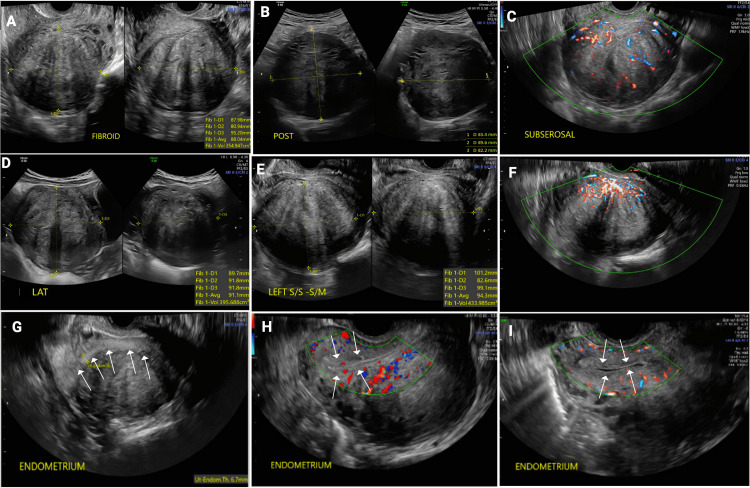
Serial annual pelvic ultrasound scans (grayscale and Doppler) performed over the five years preceding the final follow-up visit A-D represent images from five, four, three, and one years prior, and E-G represent images from three months before the final follow-up visit. A-E show the uterine fibroid as a hypoechoic mass with acoustic shadowing. C and F show positive Doppler flow, consistent with increased internal and peripheral blood flow within the fibroid. G shows the fibroid impinging on the endometrium and displacing it anteriorly (arrows). H and I are images obtained three years before the final follow-up visit; H shows a normal trilaminar endometrial lining, while I shows an anechoic intracavitary fluid accumulation within the endometrial cavity (arrows). Scale bars represent approximately 1 mm.
